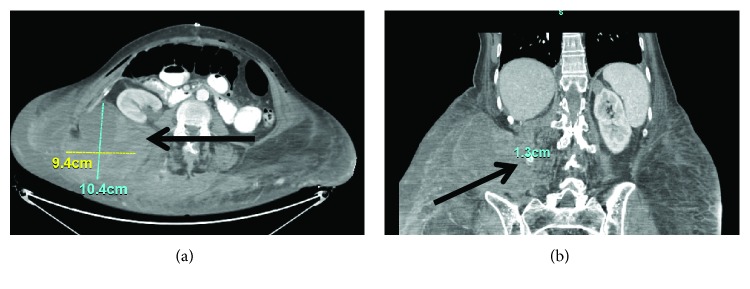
(a, b) CT. Right-sided retroperitoneal hematoma with pseudoaneurysm (arrows).
